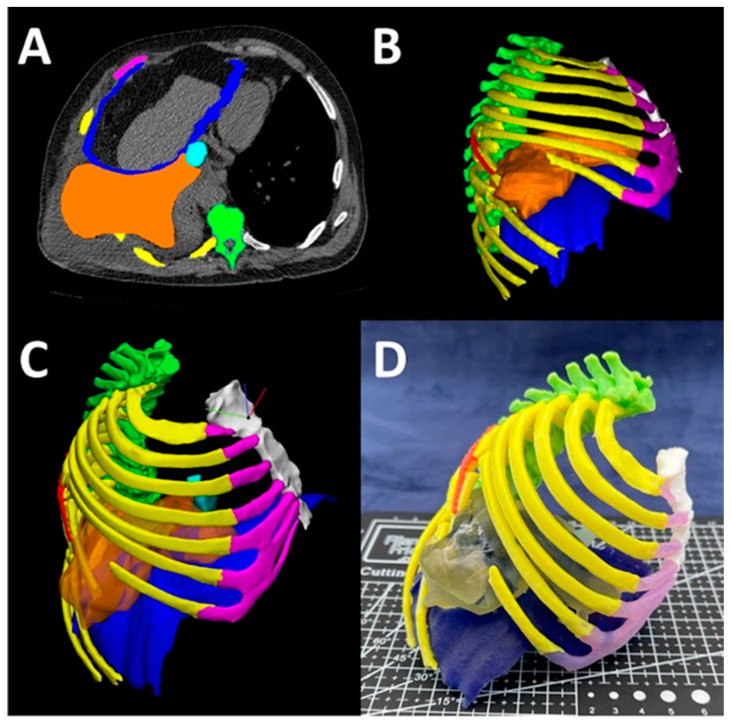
MJT 3DP anatomic model for complex chest wall and diaphragmatic hernia repair planning. The segmented anatomy included the spine (green), ribs (yellow), costal cartilage (magenta), sternum and manubrium (white), the inferior vena cava (cyan), the right hemidiaphragm (blue), the metal plate (red), and the herniated fat (orange). (A) The axial CT scan with segmented masks. (B) 3D visualization of the segmented masks. (C) The digital 3D model in Materialise 3-Matic 18 CAD software, and (D) the final full color 3D printed using MJT technology at 33% scale.
